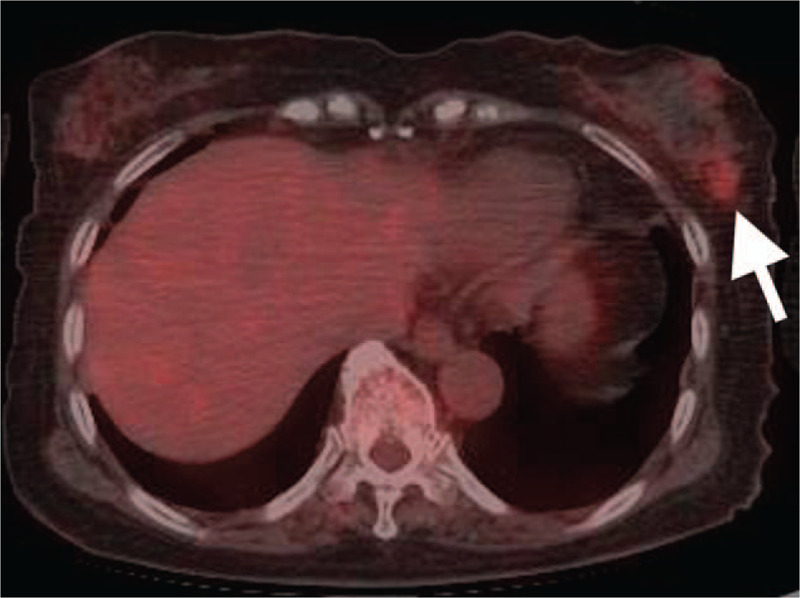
FDG PET/CT showing positive uptakes in the left breast (white arrowheads), with a standardized uptake value (SUV) of 2.9.
